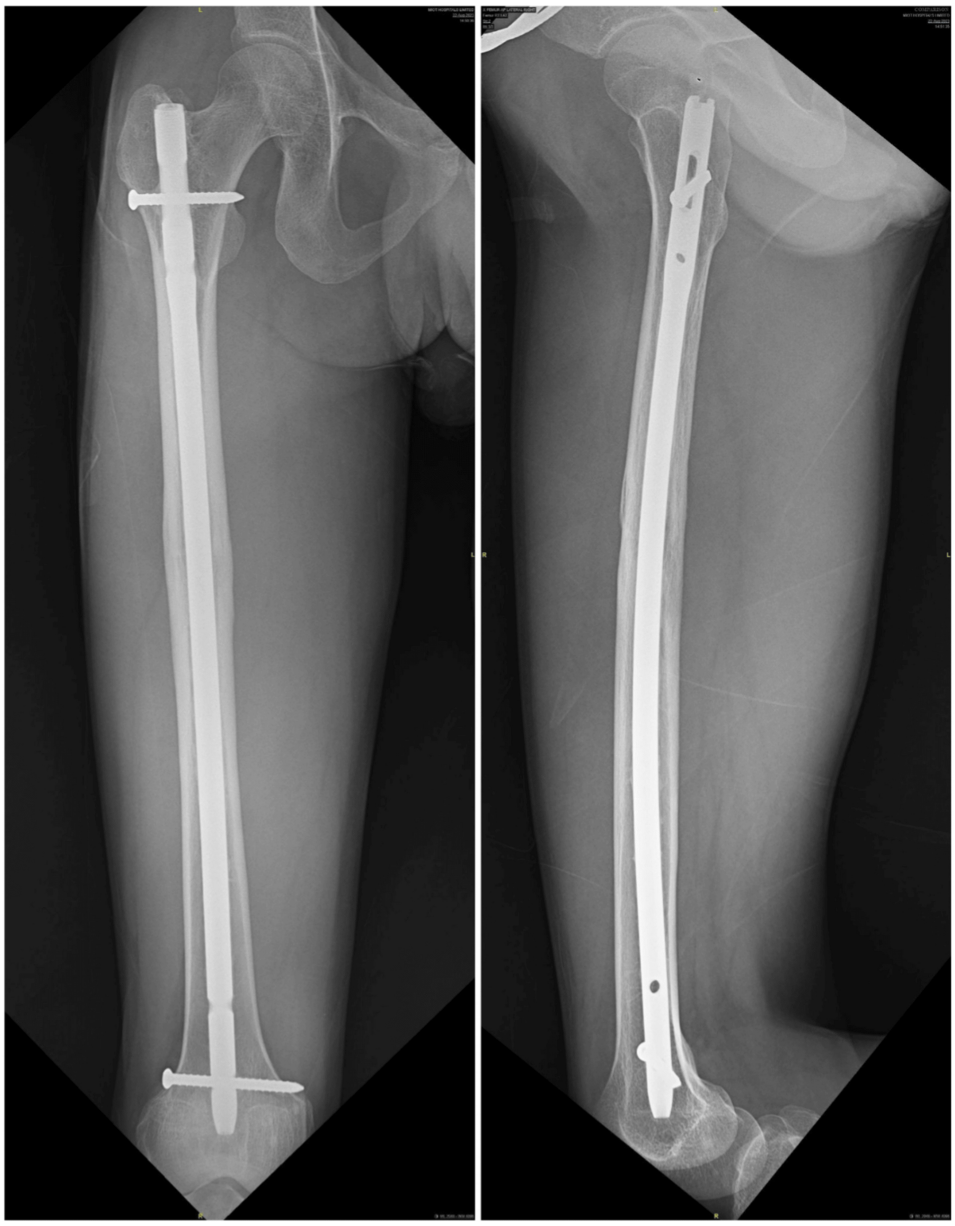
X-ray of the right femur showing healed shaft of the femur fracture, 11 years following intramedullary titanium femoral nail (10 mm in size) with one proximal locking screw in dynamic mode and two distal locking screws
